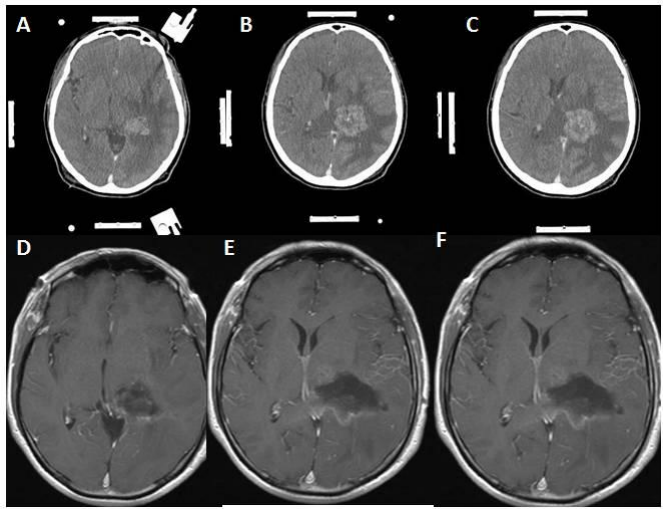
A, B, C (Table 1: Case 8, Metastatic lung tumour): Contrast-enhanced cranial tomography showing a lesion located just next to the left thalamus with large perilesional edema. Moreover, this lesion showing contrasts enhancement and heterogeneous density. The white strips at the sides of the images are the marker of the frame based the stereotaxic system. D, E, F: Gadolinium-enhanced T1 weighted axial magnetic resonance image scans taken at the postoperative period showing no residual lesion, but there is some postoperative hyperintense changing due to gliosis at the operation regions*.
